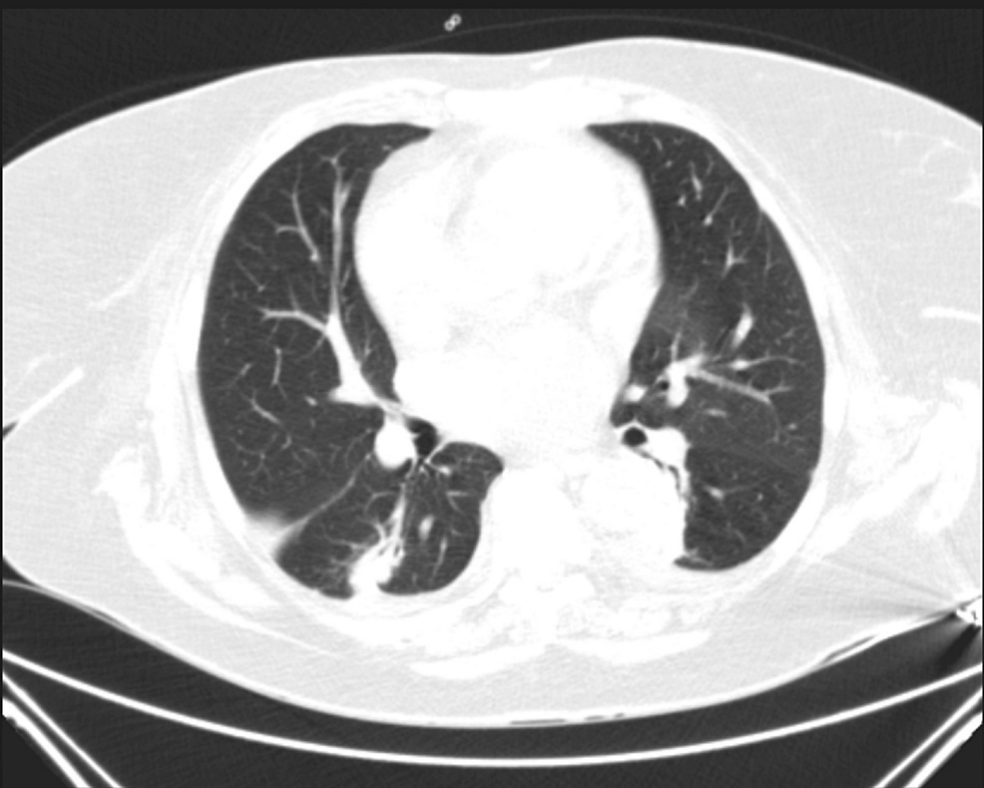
Chest computed tomography showing irregular peribronchial thickening areas and pericentimetric nodular lesions
